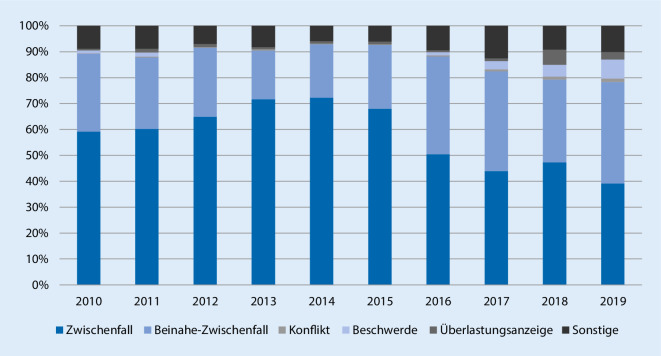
Bei 4179 Meldungen (69,5 %) waren die Versorgungsbedingungen bekannt (*n* = 4013 [66,7 %] stationär, *n* = 50 [0,8 %] ambulant, *n* = 116 [1,9 %] präklinisch). 45,2 % (*n* = 1813) der stationären Zwischenfälle wurden aus dem perioperativen Umfeld berichtet, 26,2 % (*n* = 1052) aus den Bereichen Intensivstation/IMC und Aufwachraum. 282 Fälle (7,0 %) ereigneten sich auf Normalstationen, 135 (3,4 %) in Funktions‑/Diagnostikbereichen (z. B. CT, MRT, Herzkatheter etc.). Bei 335 Fällen (8,4 %) fehlten Angaben zu Umgebungsbedingungen bzw. Ort.
